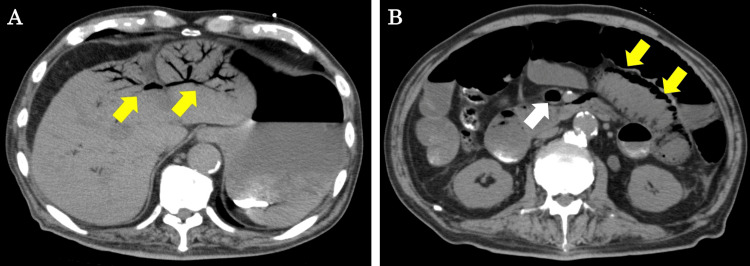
CT findings at initial examination (A) PVG can be observed mainly in the left lobe of the liver (yellow arrows). (B) Superior mesenteric venous gas (white arrow) and PI (yellow arrows) can be observed mainly in the proximal jejunum. CT, computed tomography; PVG, portal venous gas; PI, pneumatosis intestinalis
